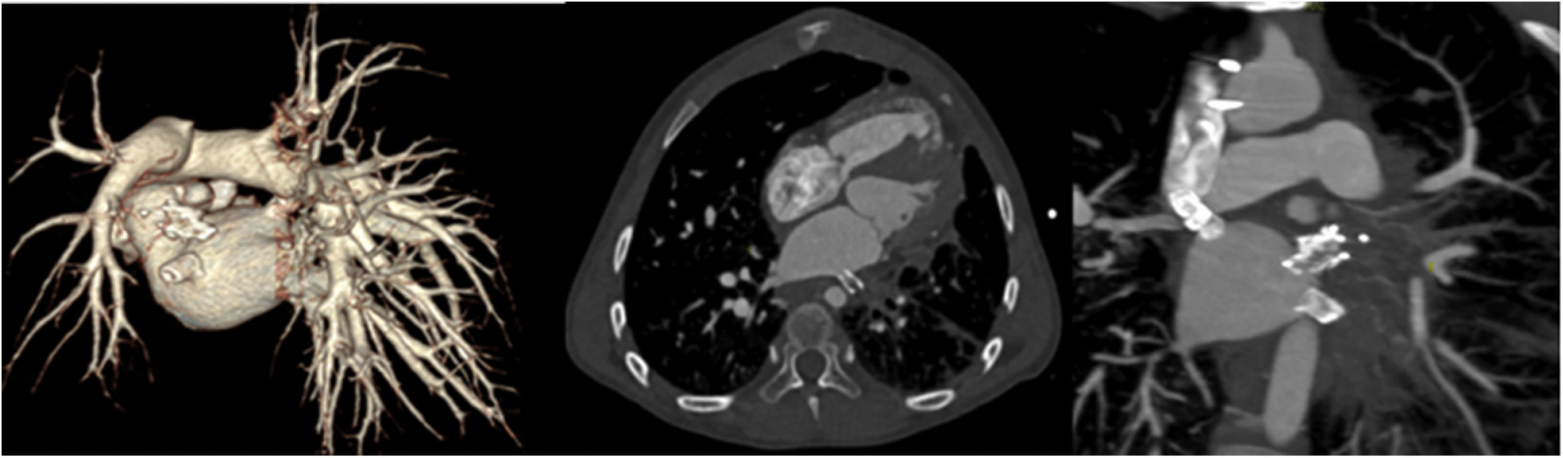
Computed Tomography Angiography Computed tomography angiography before intervention, demonstrating complete occlusion of left upper pulmonary vein and left lower pulmonary vein stents.
